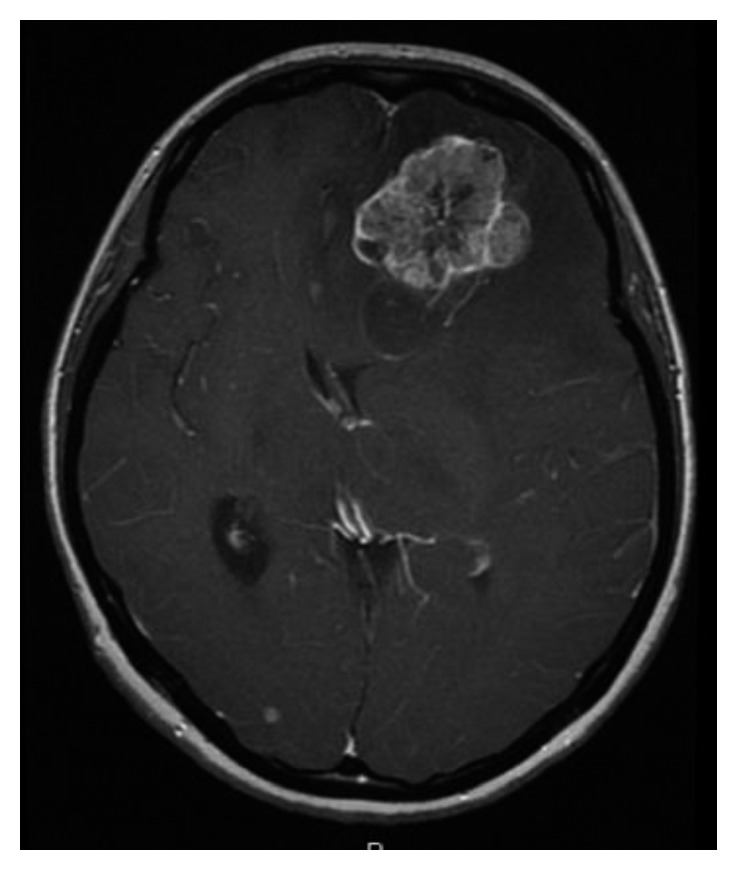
Head magnetic resonance imaging findings (T1-weighted fat suppression gadolinium-enhanced image) after 35 months of pazopanib administration. This MRI showed brain metastases with surrounding edema and a midline shift.
